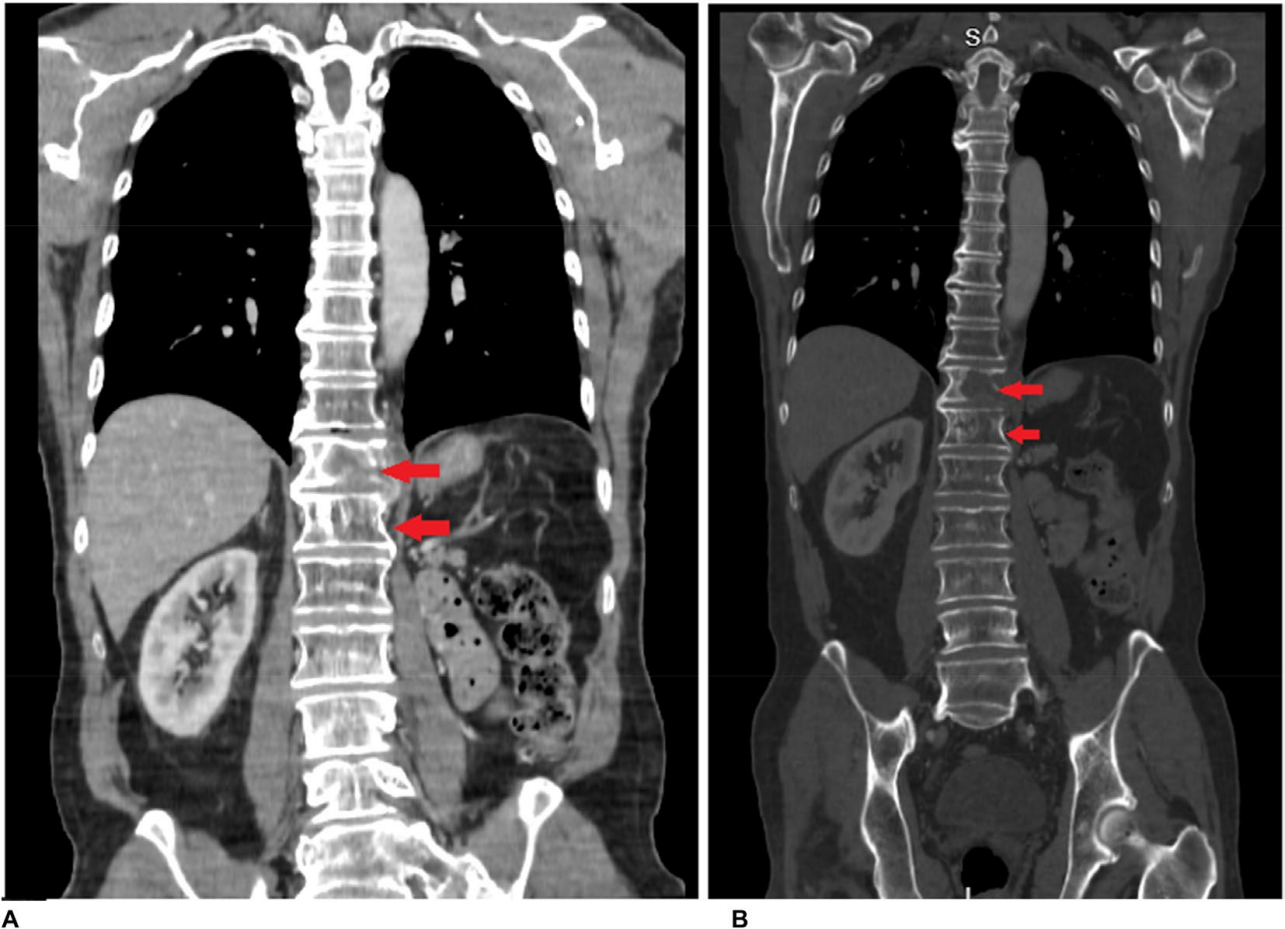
(A, B) MRI of the thoracic spine reveals hypointense vertebral lesions on T1‐weighted imaging and hyperintense or mixed intensity on T2/STIR sequences. These findings suggest bone marrow infiltration with tumor involvement, with a potential for epidural extension, increasing the risk of spinal cord compression.
